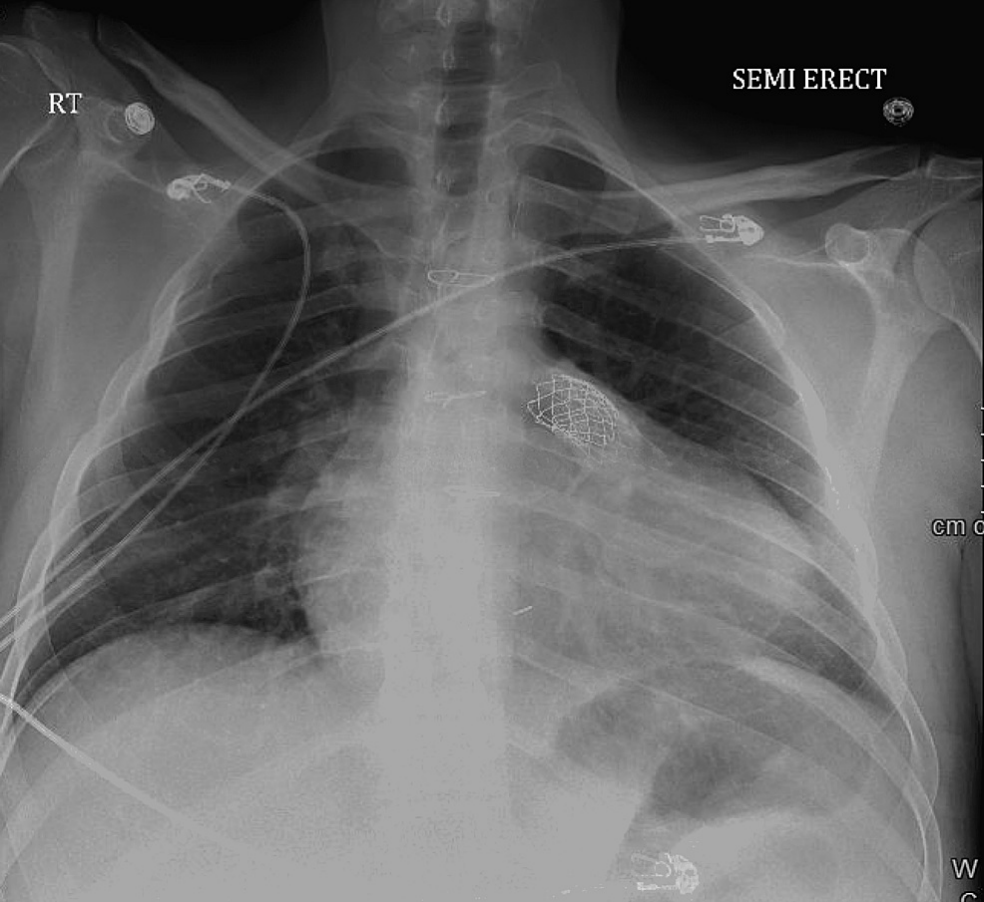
Chest radiograph demonstrating no acute cardiopulmonary process and stent in the RVOT RVOT: right ventricular outflow tract
